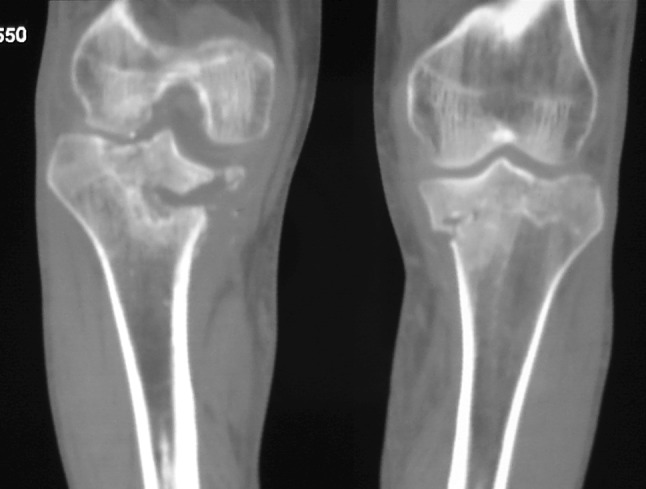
Computed tomography (CT) of both knees. The computed tomography of both knees showed bilateral tibial plateau fracture on medial side, fragmentation, and destruction coalescence
